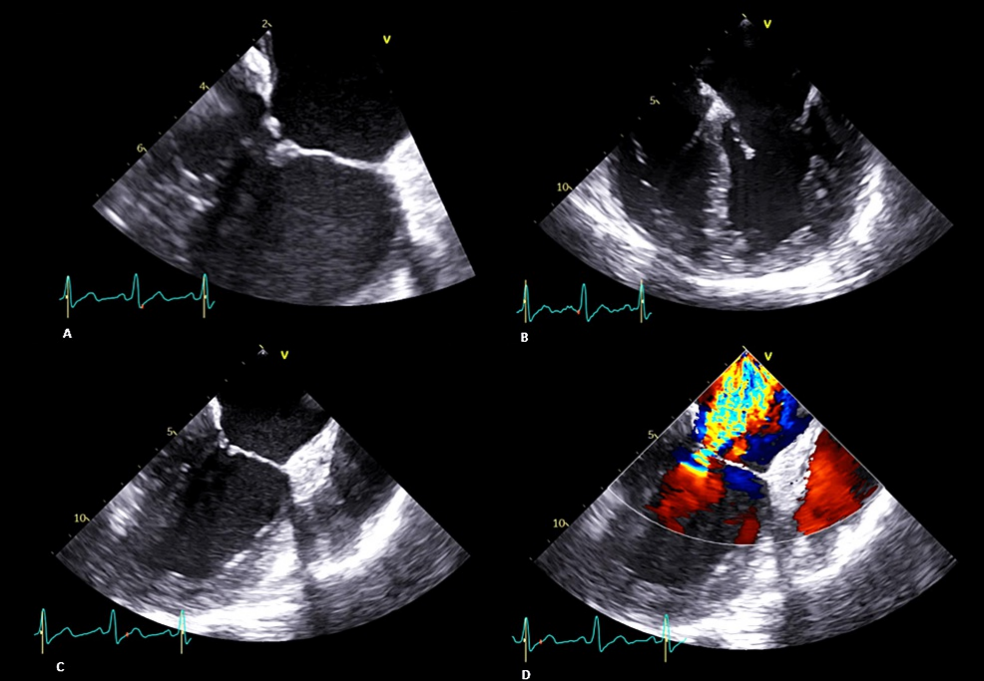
Transesophageal echocardiogram images A, B, and C: Thickened distal ends of the mitral valve leaflets; D: Moderate to severe mitral regurgitation
